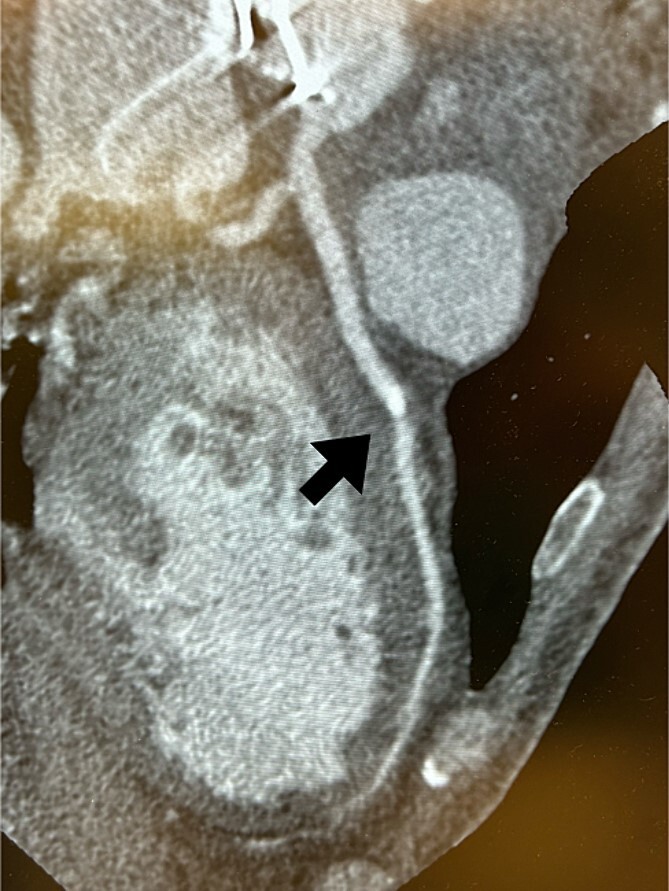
Coronary CT scans revealed a high-density area (arrow) in the LAD artery.
